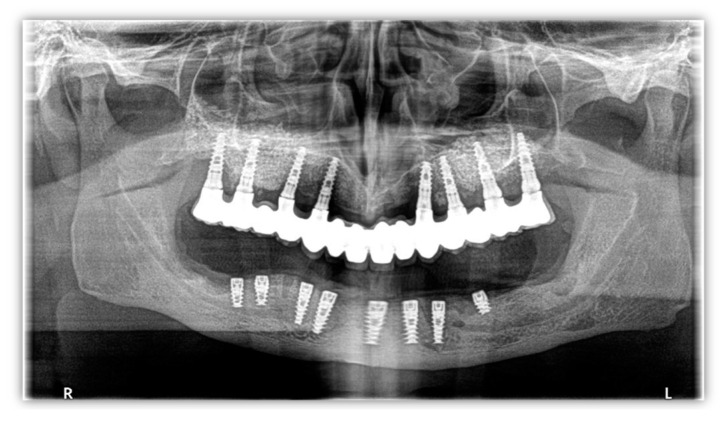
Orthopantomography image from 2014.
